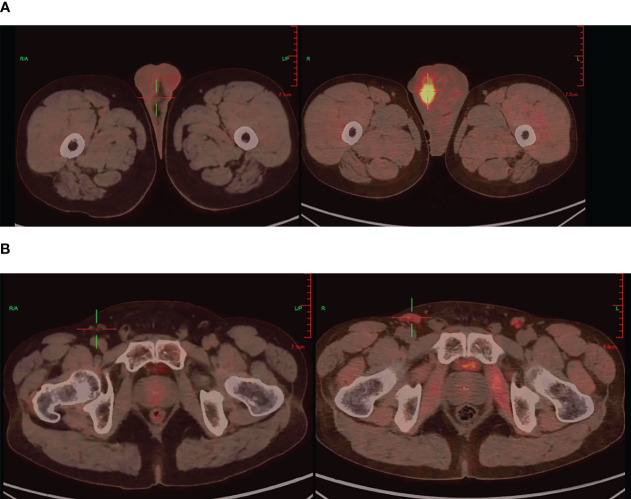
PET/CT imaging: Testicular before targeted therapy (right), after targeted therapy (left) (A), Inguinal lymph nodes before targeted therapy (right), after targeted therapy (left) (B).
